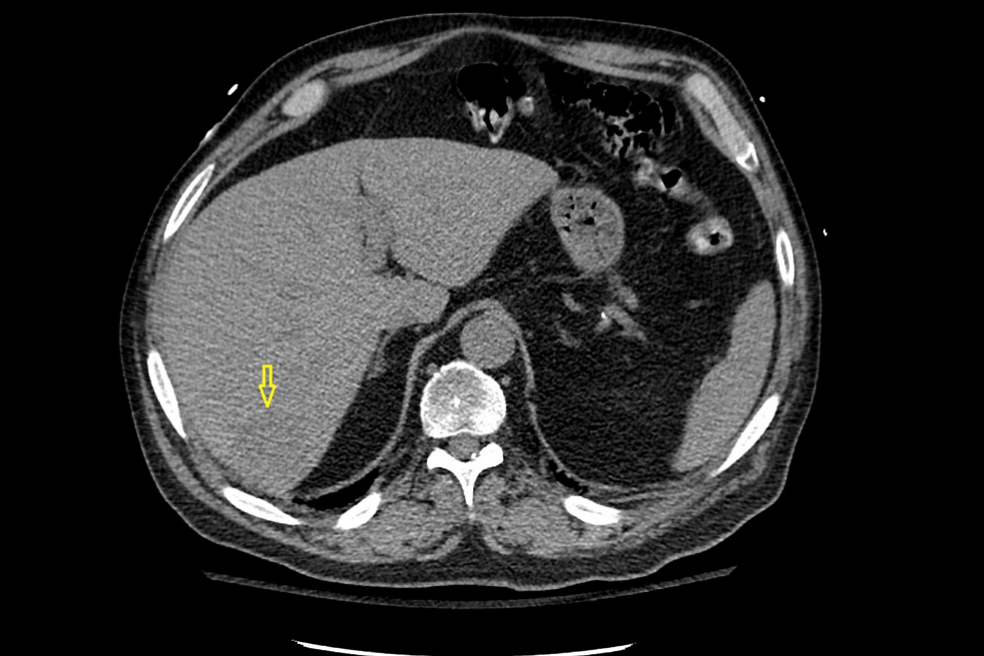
CT liver showing nodule in disseminated histoplasmosis (arrow) CT: computed tomography
